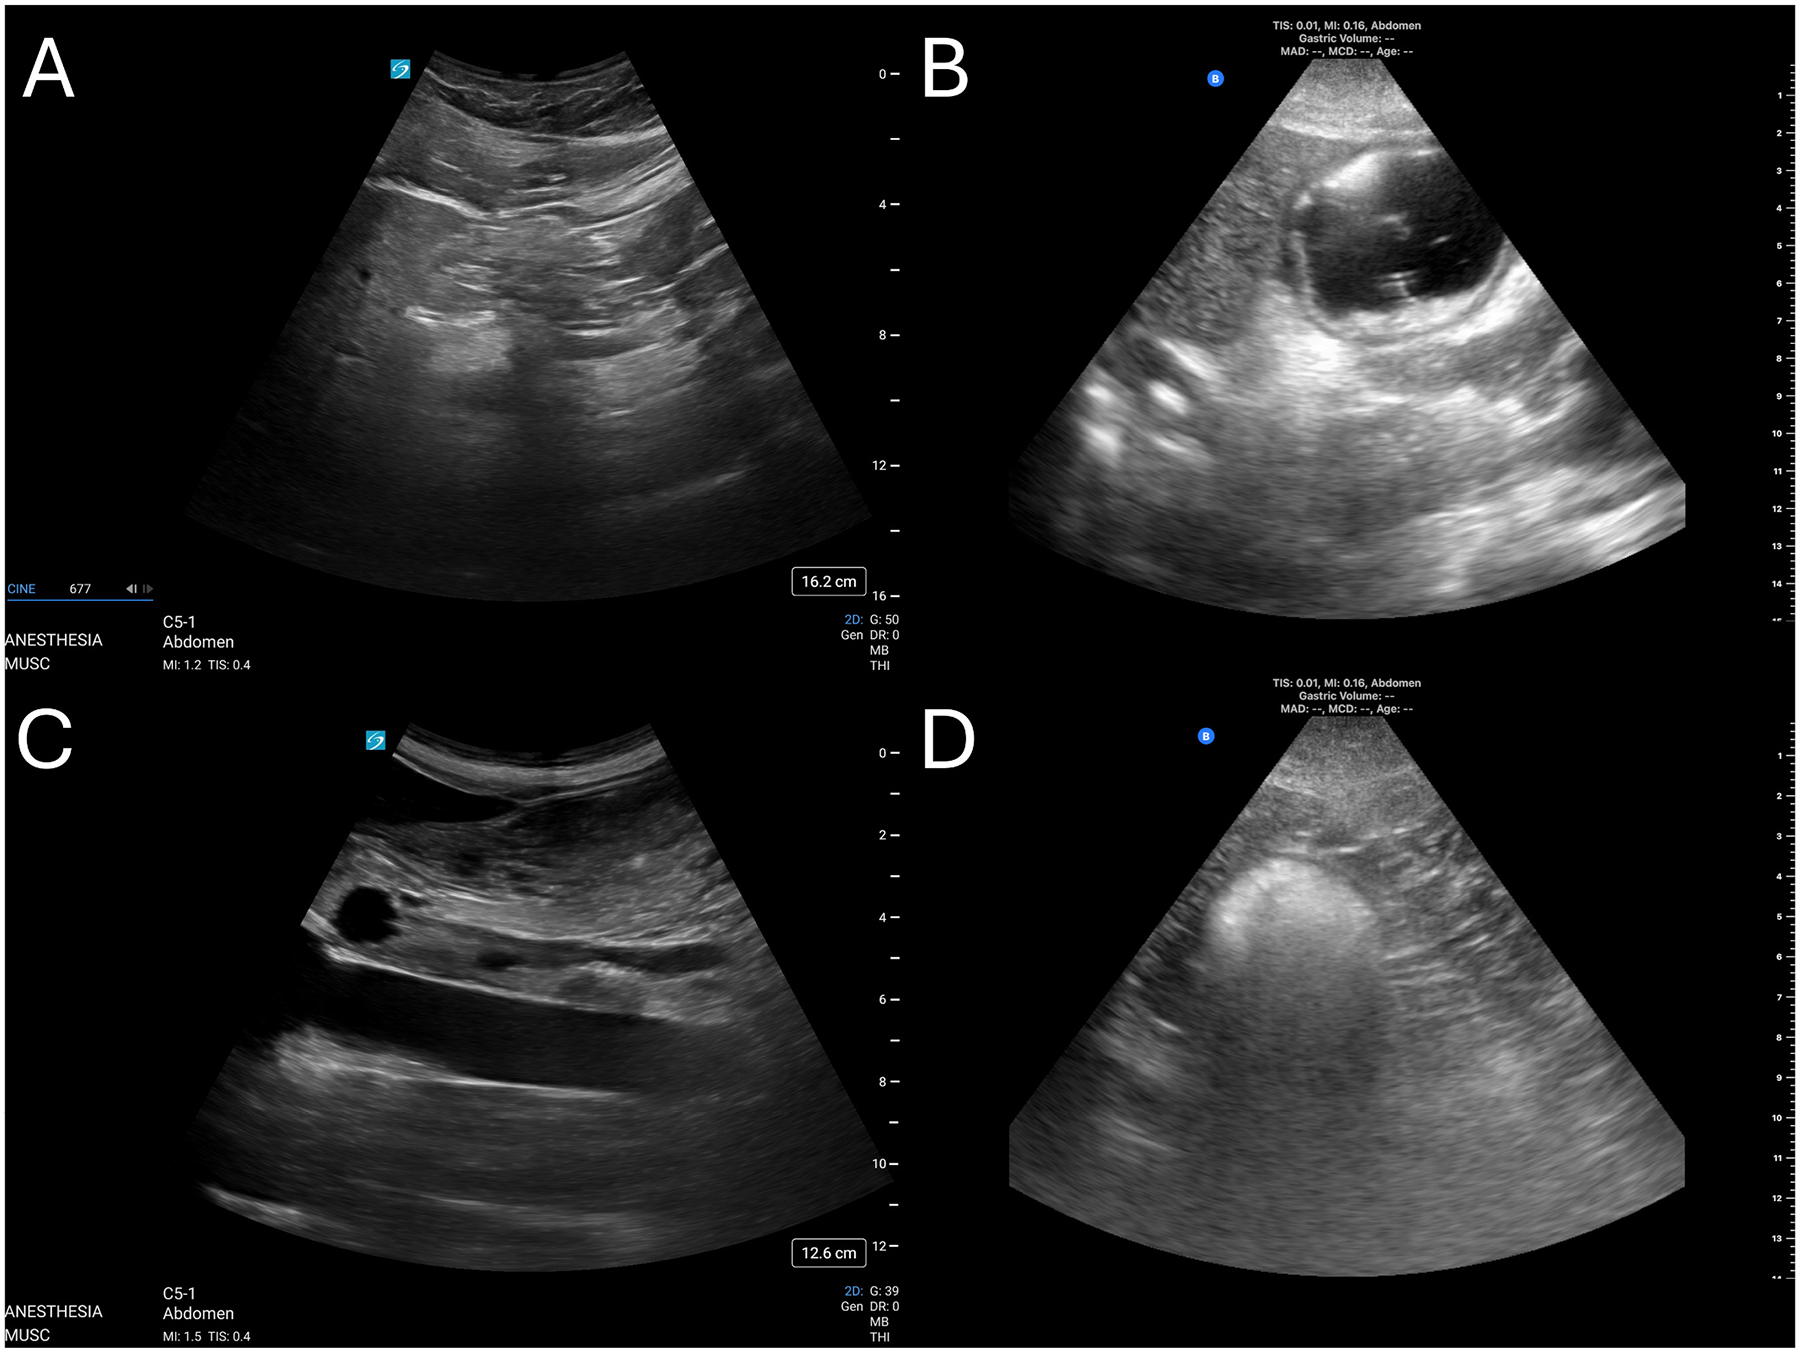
Ultrasound images of qualitative antral appearances in the right lateral decubitus position. (A) Empty antrum, fully collapsed. (B) Distended antrum containing clear liquids. (C) Greatly distended antrum containing thick liquids/late stage solids. (D) Antrum with recent ingestion of solids (“frosted glass” appearance).
